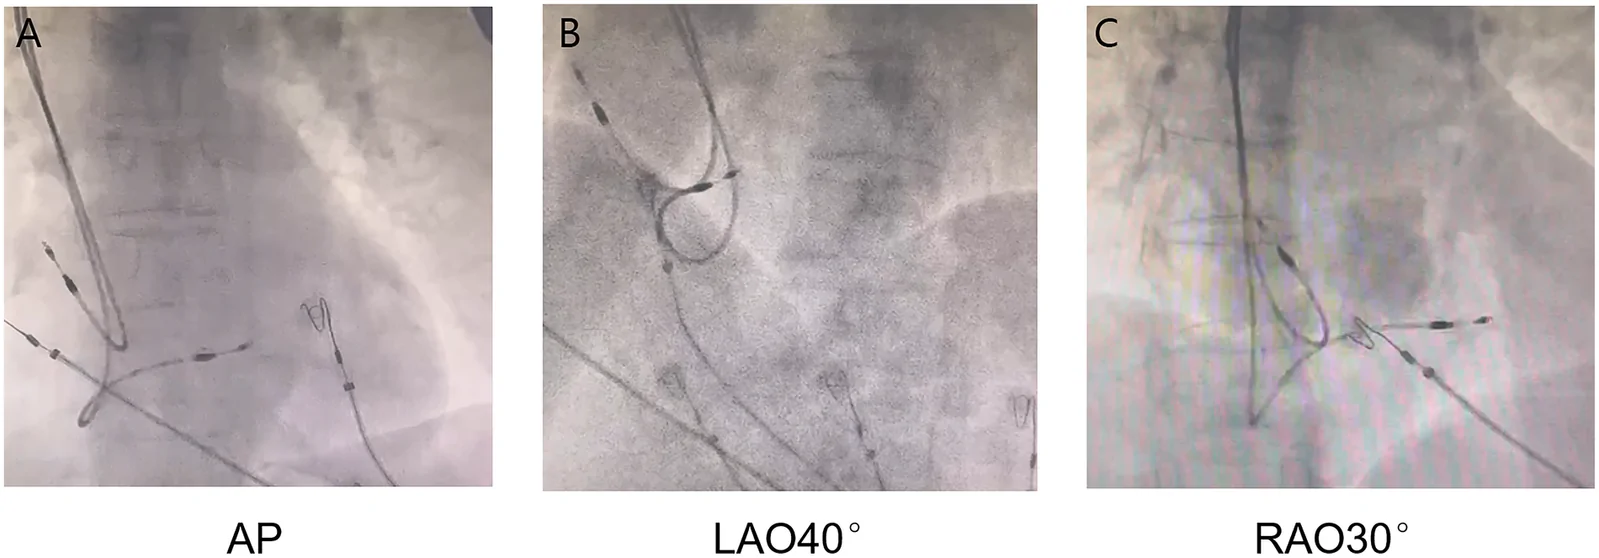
Fluoroscopy of leads. Representative x-ray images were acquired in anteroposterior (A), left anterior oblique 40° (B), and right anterior oblique 30°views (C), respectively.
